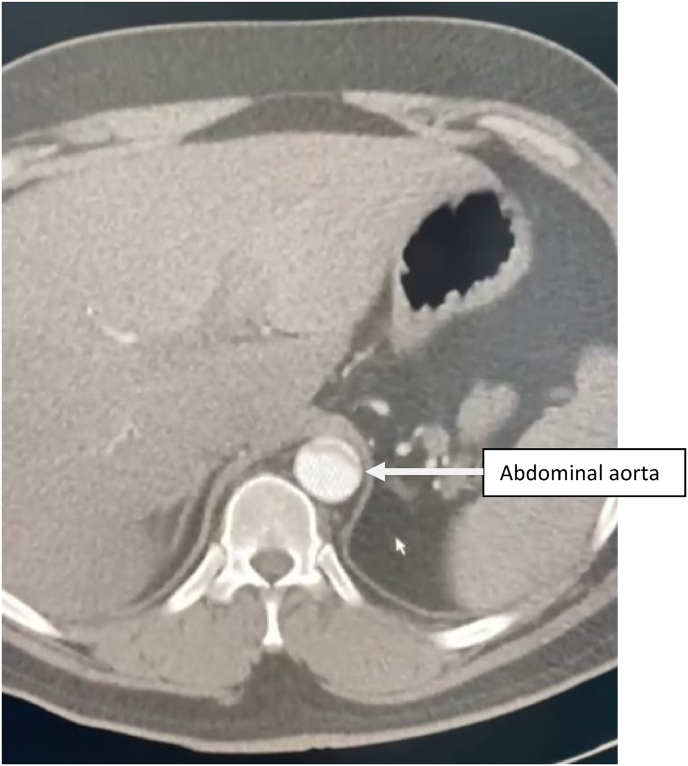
CT scan image showing the extension of the dissection to the abdominal aorta.
